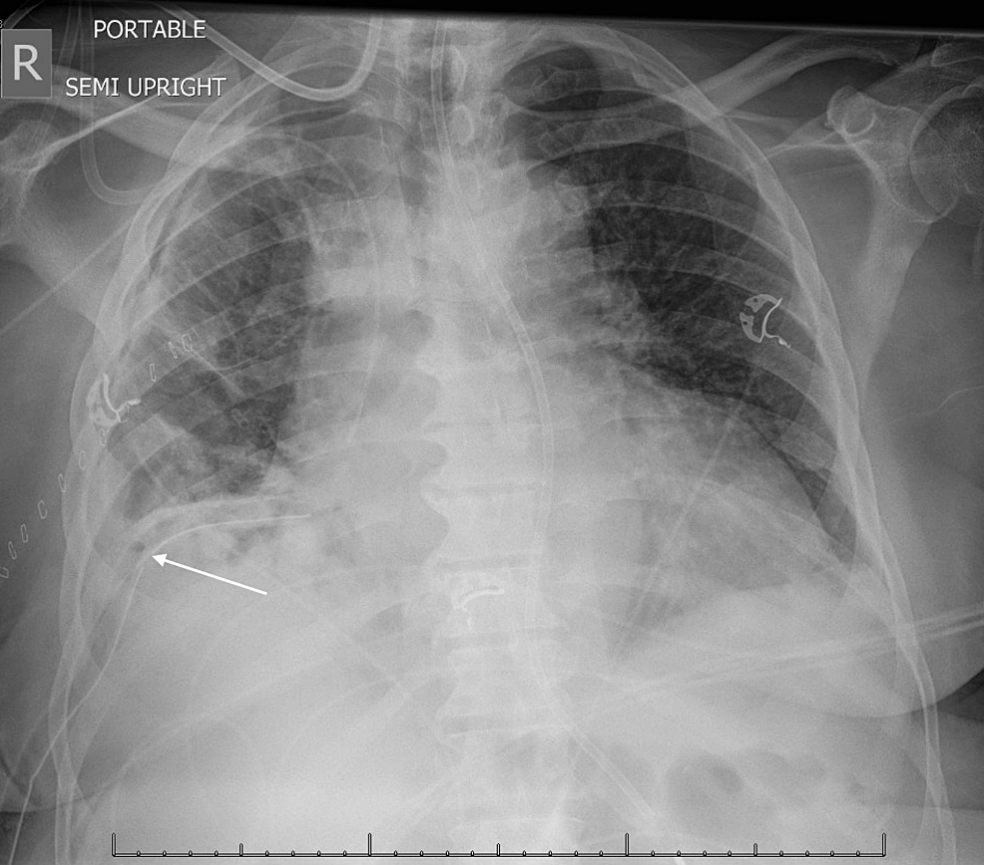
Post-surgery chest X-ray showing improvement in multifocal lung opacities with the chest tube (arrow) in place
